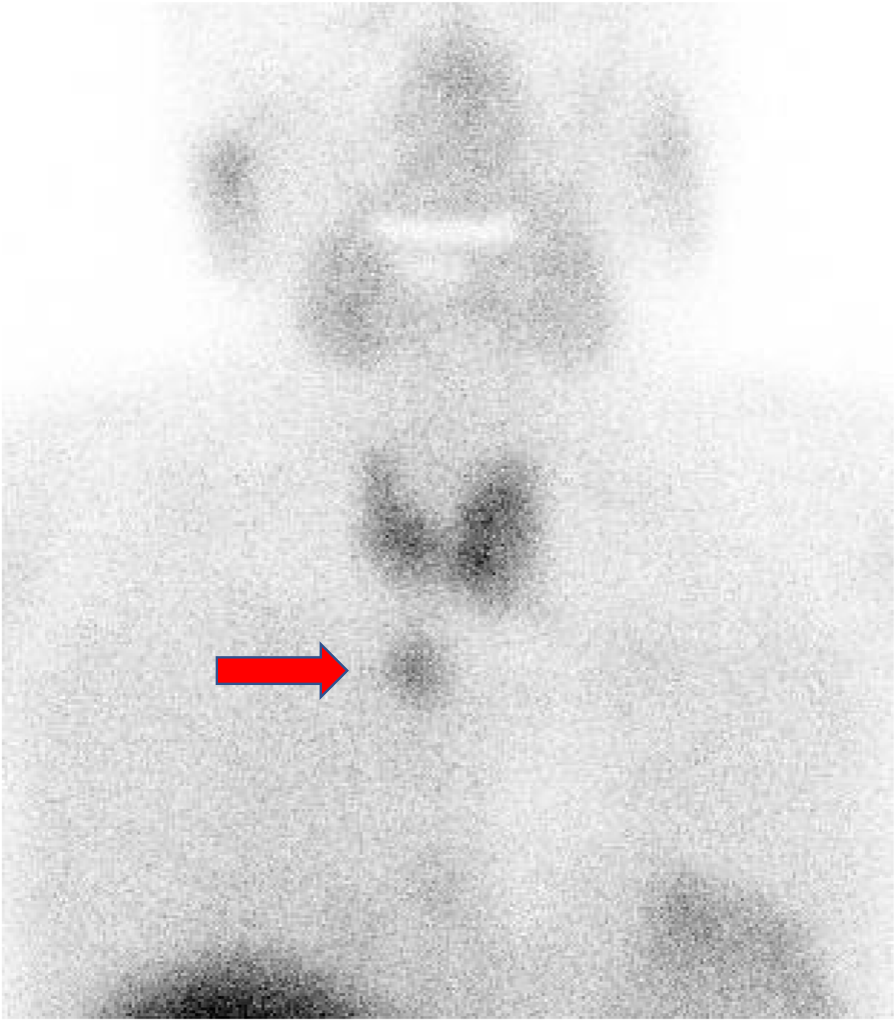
Technetiuim-99m methoxy isobutyl isonitrile scintigraphy showing an area of increased mediastinal uptake during the early phase
